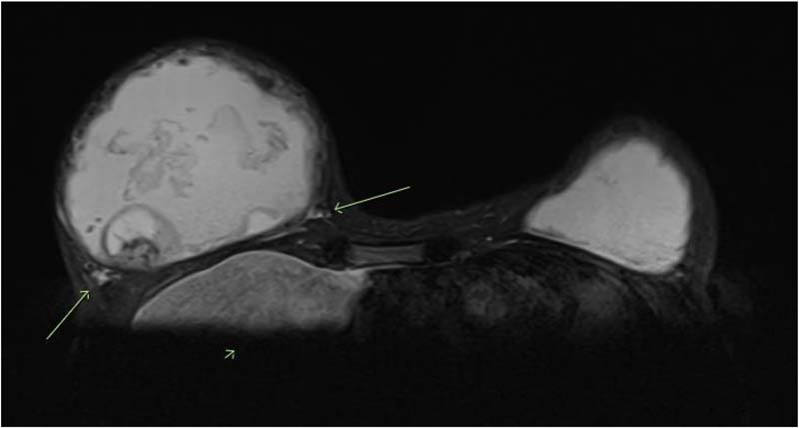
T2 weighted MRI of bilateral breasts—showing irregular borders of the fibrous capsule, heterogeneous material within the capsule, an intracapsular lesion, and extracapsular signal, suggestive of intracapsular and extracapsular breast implant rupture.
